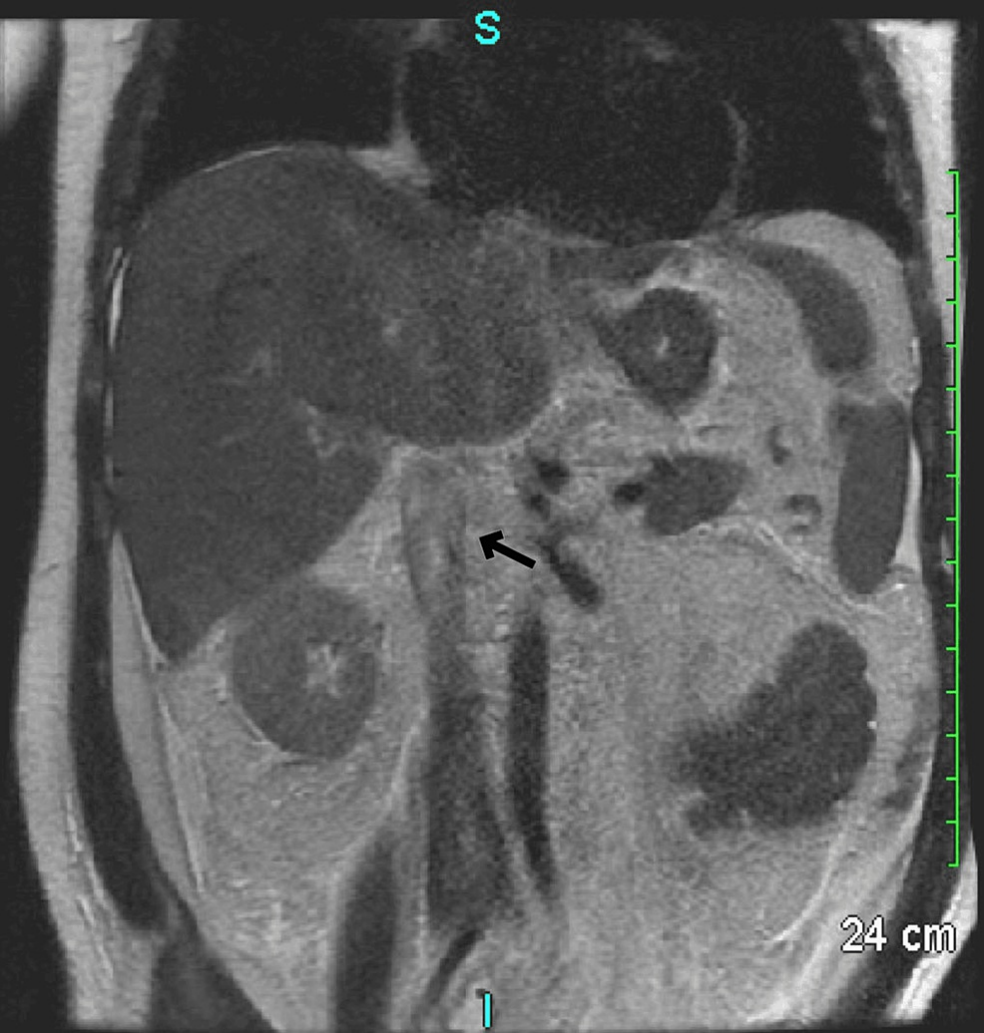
MRI Abdomen Arrow pointing toward a filling defect in the inferior vena cava.
